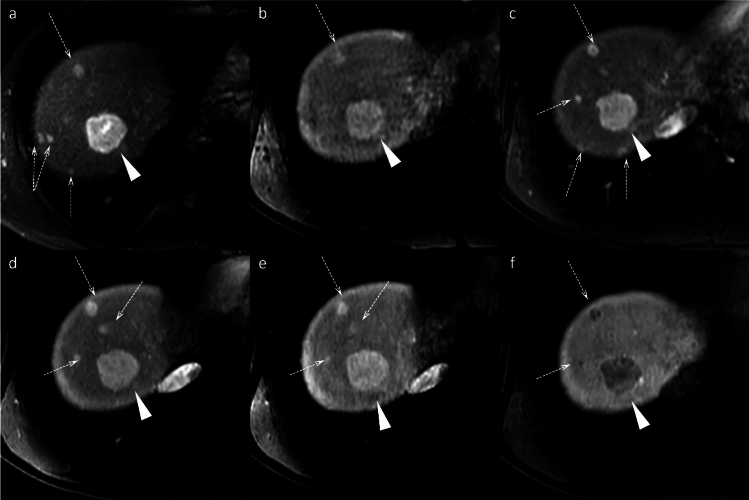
Inflammatory hepatocellular adenomas in a 21-year-old man with glycogen storage disease type I. A mass (arrowheads) located in segment 8 shows clear hyperintensity on fat-suppressed T2-weighted image, with an atoll sign evident (a). The mass displays hyperintensity on pre-contrast image (b), non-rim arterial phase hyperenhancement (c) without portal venous washout (d), and hyperenhancement on the transitional phase image (e). The mass shows homogeneous hypointensity on the hepatobiliary phase image (f). Multiple additional small nodules (arrows), suspicious for hepatocellular adenoma, are also noted throughout images (a–f)
